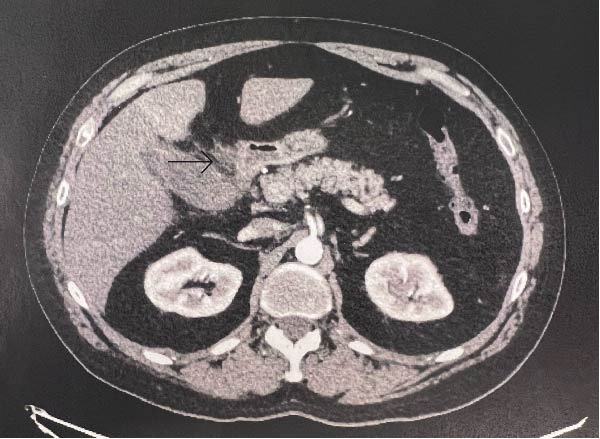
CT scan showing pericholecystic fat stranding (arrow).
